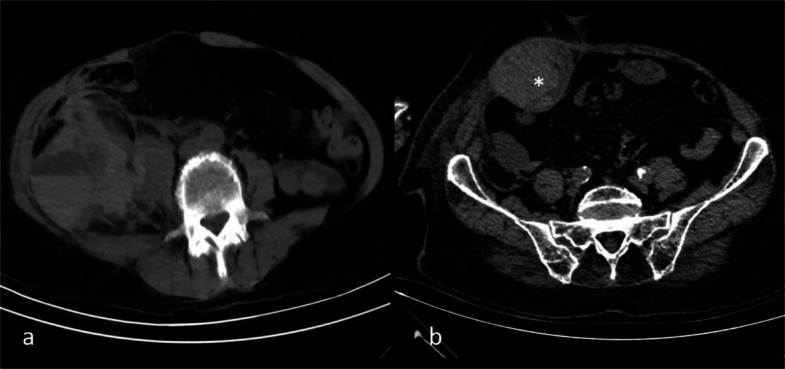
Non-contrast computed tomography showing the hematocrit effect with fluid–fluid levels in a right iliopsoas hematoma (a) and the sentinel clot sign, with hyperdense acute clot (asterisk) indicating proximity to active bleeding, in a right rectus sheath hematoma (b)
